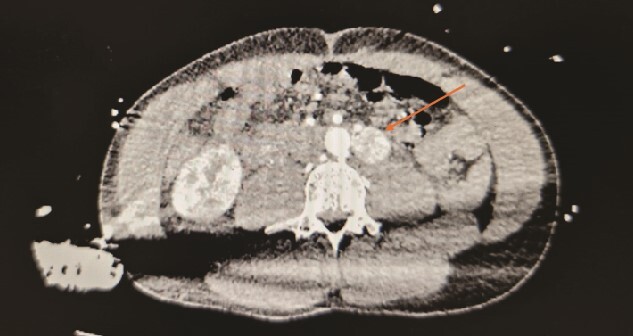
Contrasted CT in arterial phase, in the axial plane, showed a contrast enhanced, hyperdense, heterogenous lesion located over the left para-aortic region, measuring ⁓2 × 2 × 3 cm with no septations or peripheral rim enhancement.
